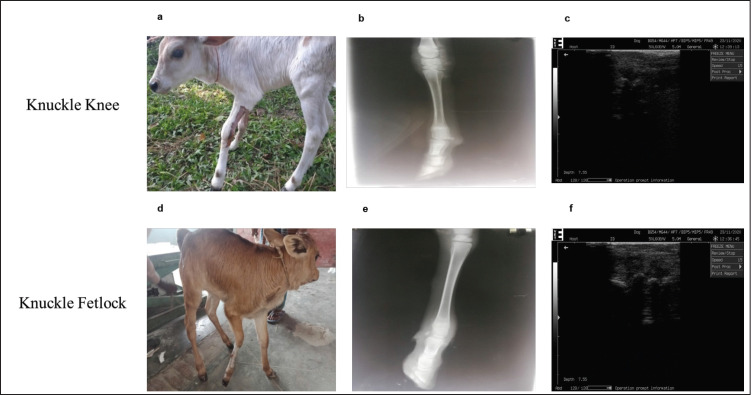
Postoperative clinical, radiographic, and ultrasonographic examination in calves a) clinical examination in knuckle knee b) radiographic examination in knuckle knee c) ultrasonographic examination in knuckle knee d) clinical examination in knuckle fetlock e) radiographic examination in knuckle fetlock f) ultrasonographic examination in knuckle fetlock.
